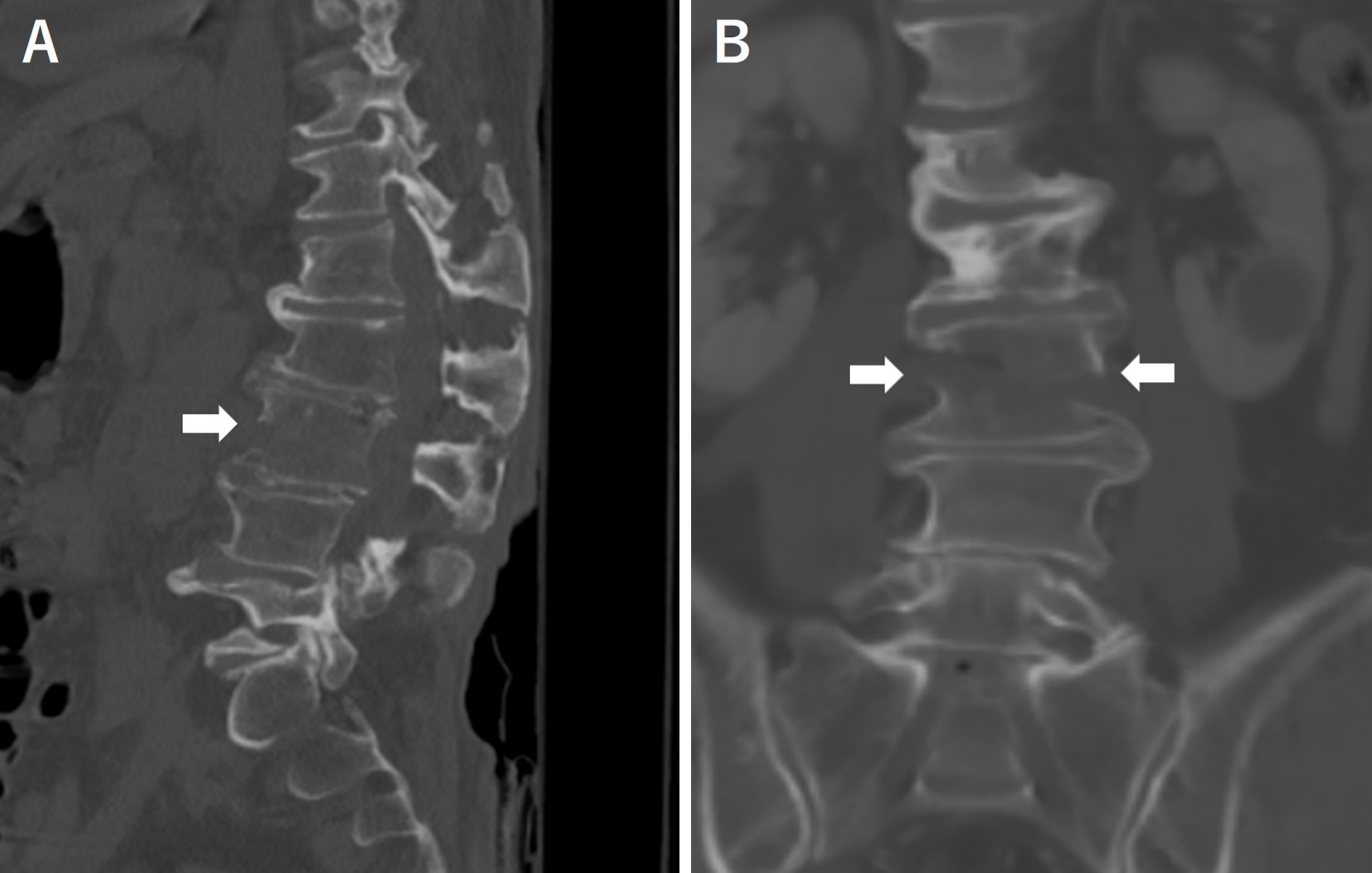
Sagittal (A) and coronal (B) slices of plain computed tomography revealed diffuse idiopathic skeletal hyperostosis and a fracture of the third lumbar vertebra.
